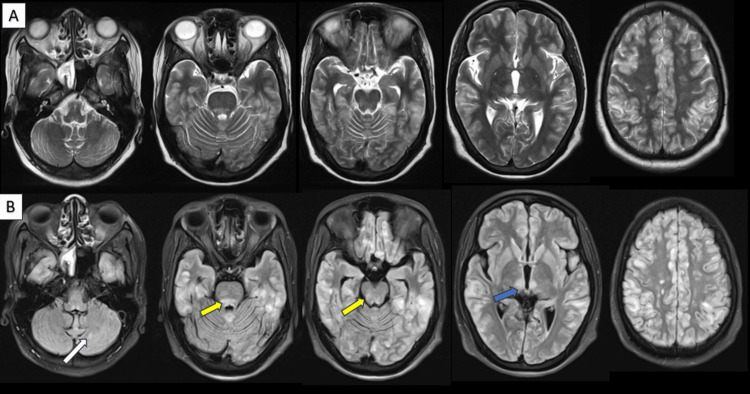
Axial T2W (A) and FLAIR (B) brain MRI. A 21-year-old female with chronic kidney disease presented with headaches and seizures. Axial brain MRI showed T2/FLAIR hyperintensities in the subcortical white matter of the left cerebellar hemisphere (white arrow), brain stem (yellow arrow), ​​​​​​​bilateral thalami (blue arrow), and bilateral cerebral hemispheres. T2W: T2-weighted; FLAIR: fluid-attenuated inversion recovery.
